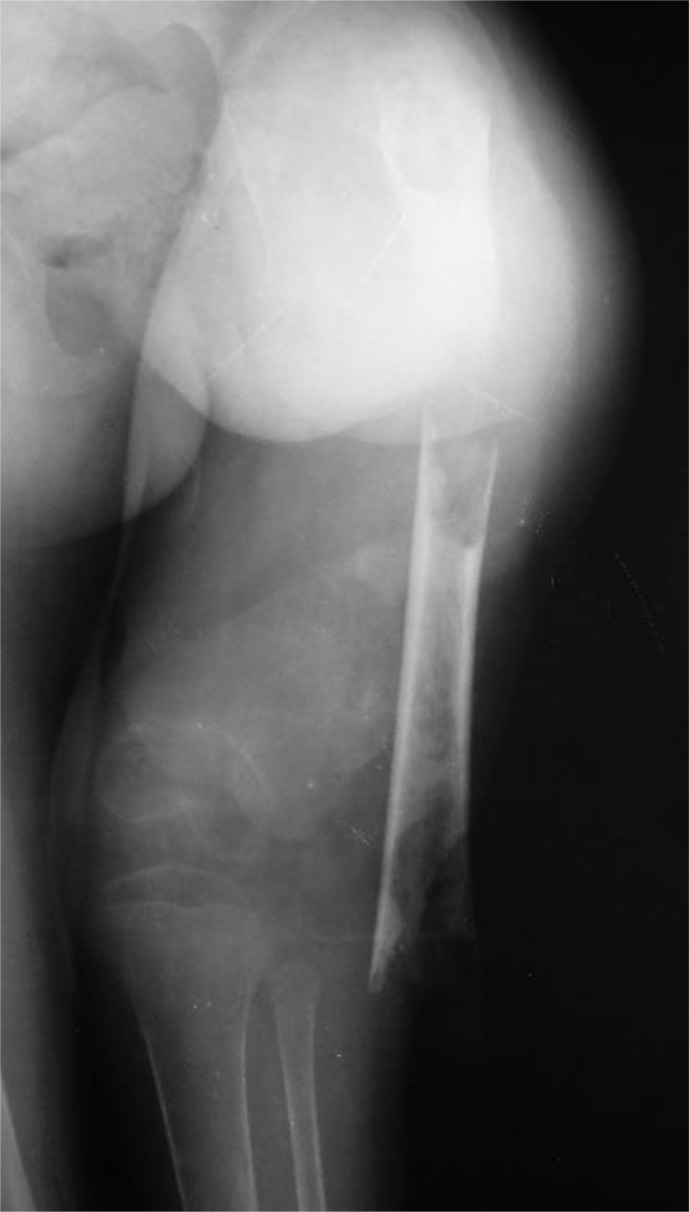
Radiograph of a 5-year-old child who presented with an extruded
